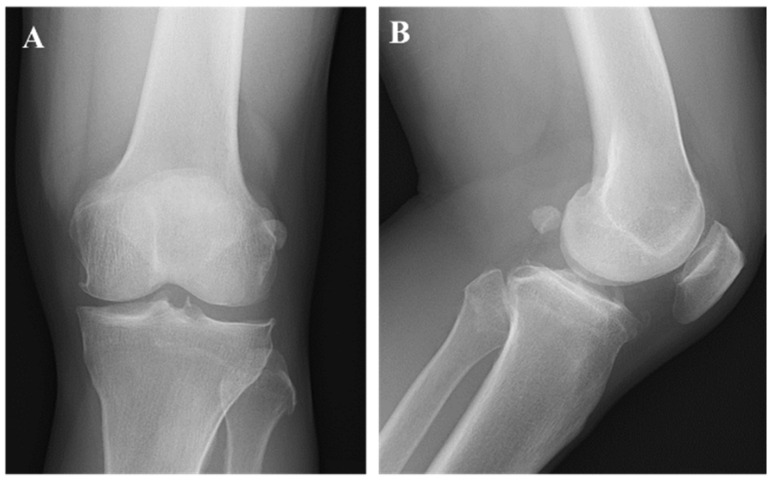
A 66-year-old man presented with a 10-year history of left knee pain. He had been diagnosed as having osteoarthritis of the knee at a local clinic and managed conservatively. However, over the past 5 years, the pain became more frequent, and he also noticed a palpable mass, prompting referral to our hospital for further evaluation. On physical examination, a soft, elastic mass was palpable around the left knee, with normal overlying skin color. Marked tenderness was present, but Tinel’s sign was absent. Joint effusion was noted, although there was no warmth. The range of motion of the knee was 0–120°, and despite pain, the patient was able to ambulate without assistance. His past medical history included surgeries for gastric and colorectal cancers, with no evidence of recurrence or metastasis at the time of our first evaluation. Laboratory tests indicated no elevation of inflammatory markers. Plain radiographs of the knee (A,B) demonstrated Kellgren–Lawrence grade 2 osteoarthritis, without soft tissue calcification or any obvious bony abnormalities.
